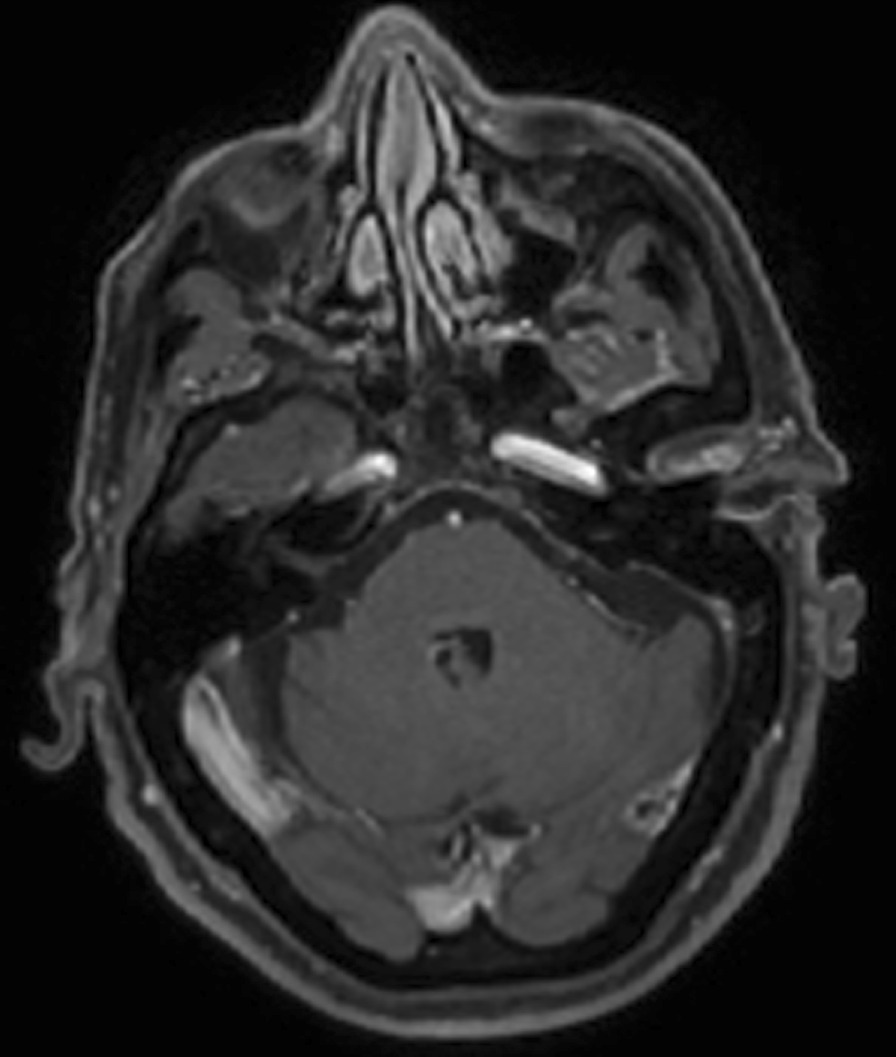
Complete resolution of radiological findings shown on previous studies were observed on MRI performed one year later. No soft tissue mass, bone infiltration or extension to posterior fossa were noted
